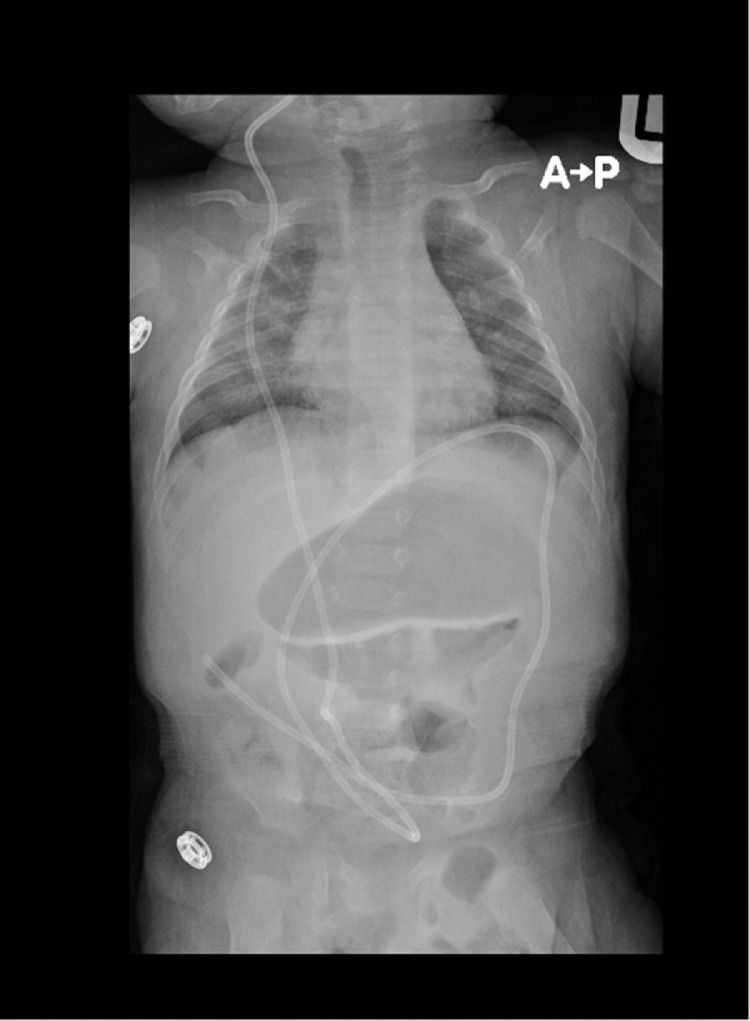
Abdominal radiograph postreduction.
